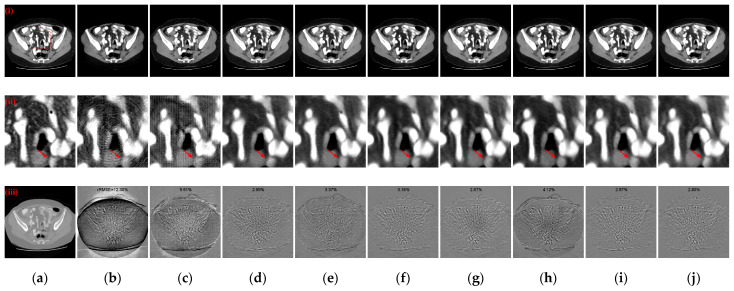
Qualitative comparative results of different ablation models. (a) The reference image; (b) baseline; (c) Model 2; (d) Model 3; (e) Model 4; (f) Model 5; (g) Model 6; (h) Model 7; (i) Model 8; (j) Ours. (i)–(iii) are reconstructed CT images, enlarged ROIs, and absolute difference images, respectively. The display window for the reconstructed CT images and enlarged ROIs is [−160, 240] HU; the display window for absolute difference images is [−180, 180] HU.
